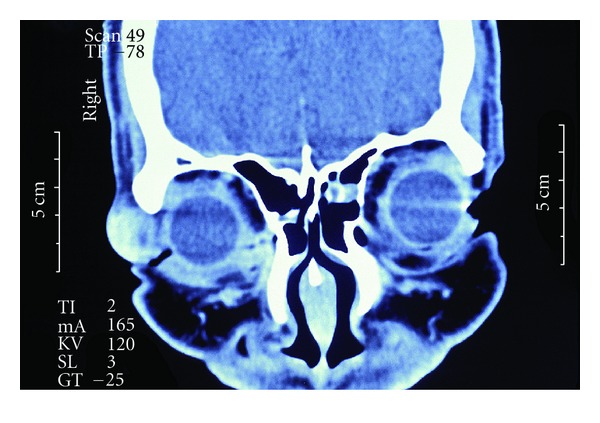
CT scan of right orbital rim mass.
